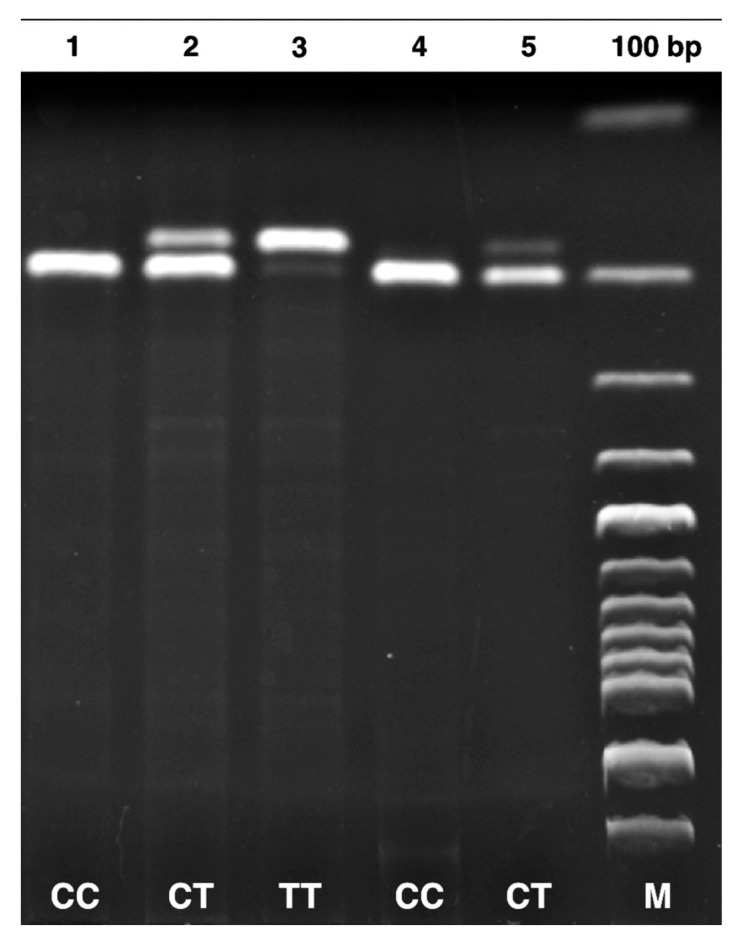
Gel electrophoresis of PCR–amplified product of MTHFR C677T gene polymorphism. CC (198 bp), TT (175 bp), CT (175–198 bp), and M (100 bp DNA molecular weight marker (abm, Katalog No: G193)).
